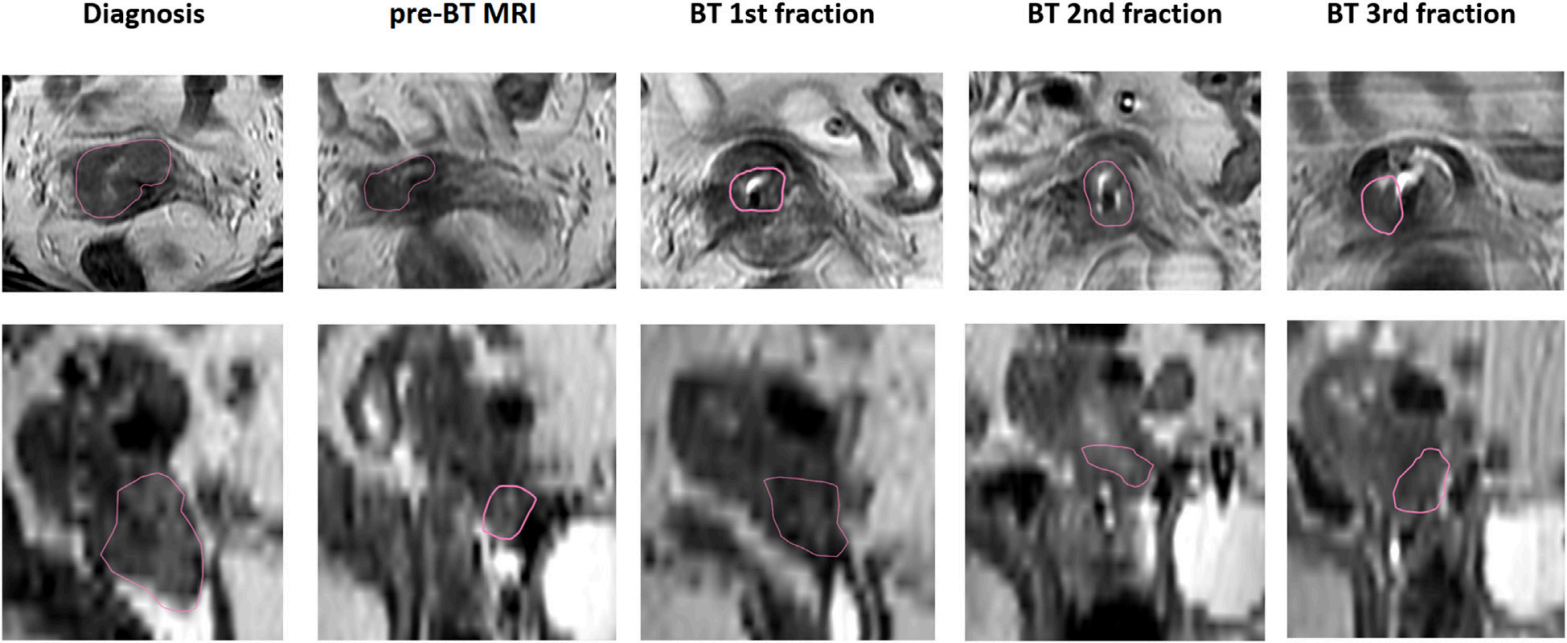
MRI-based GTV evaluation during treatment, transversal and sagittal views (red contour indicates the GTV).
